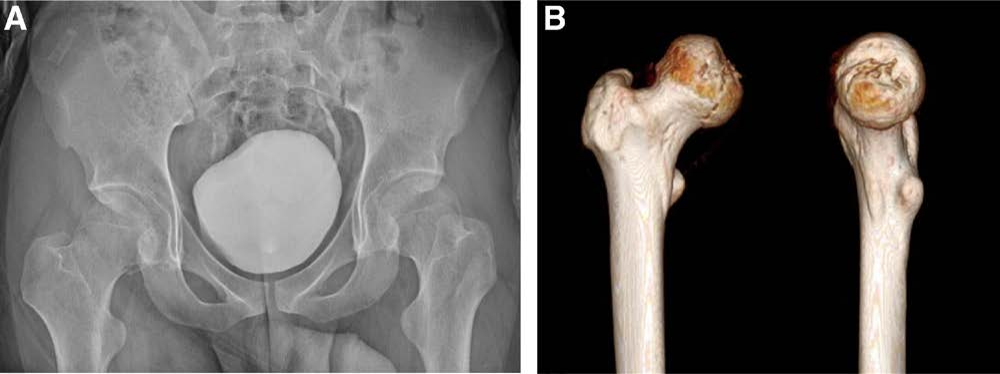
(A): Anteroposterior hip x-ray after reduction shows correct positioning of the femoral head. (B): 3-dimension reconstruction image of the femoral head showing a large defect in the weight-bearing surface.
